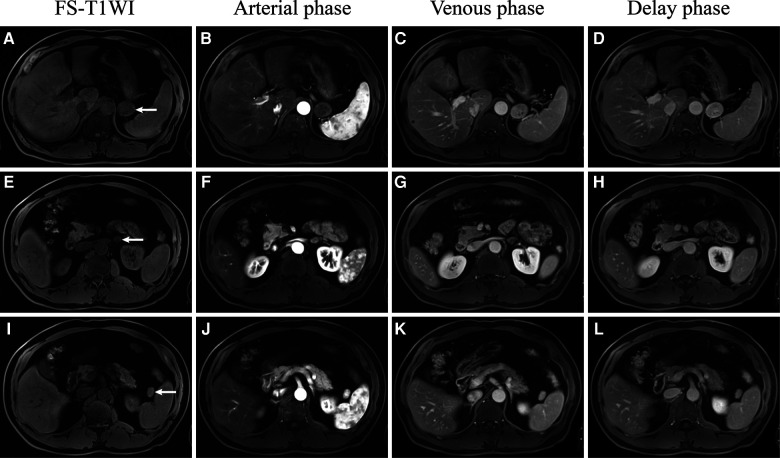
Dynamic gadolinium-enhanced MRI of the retroperitoneal ectopic pancreas (A–D), adrenal adenoma (E–H), and accessory spleen (I–L).
